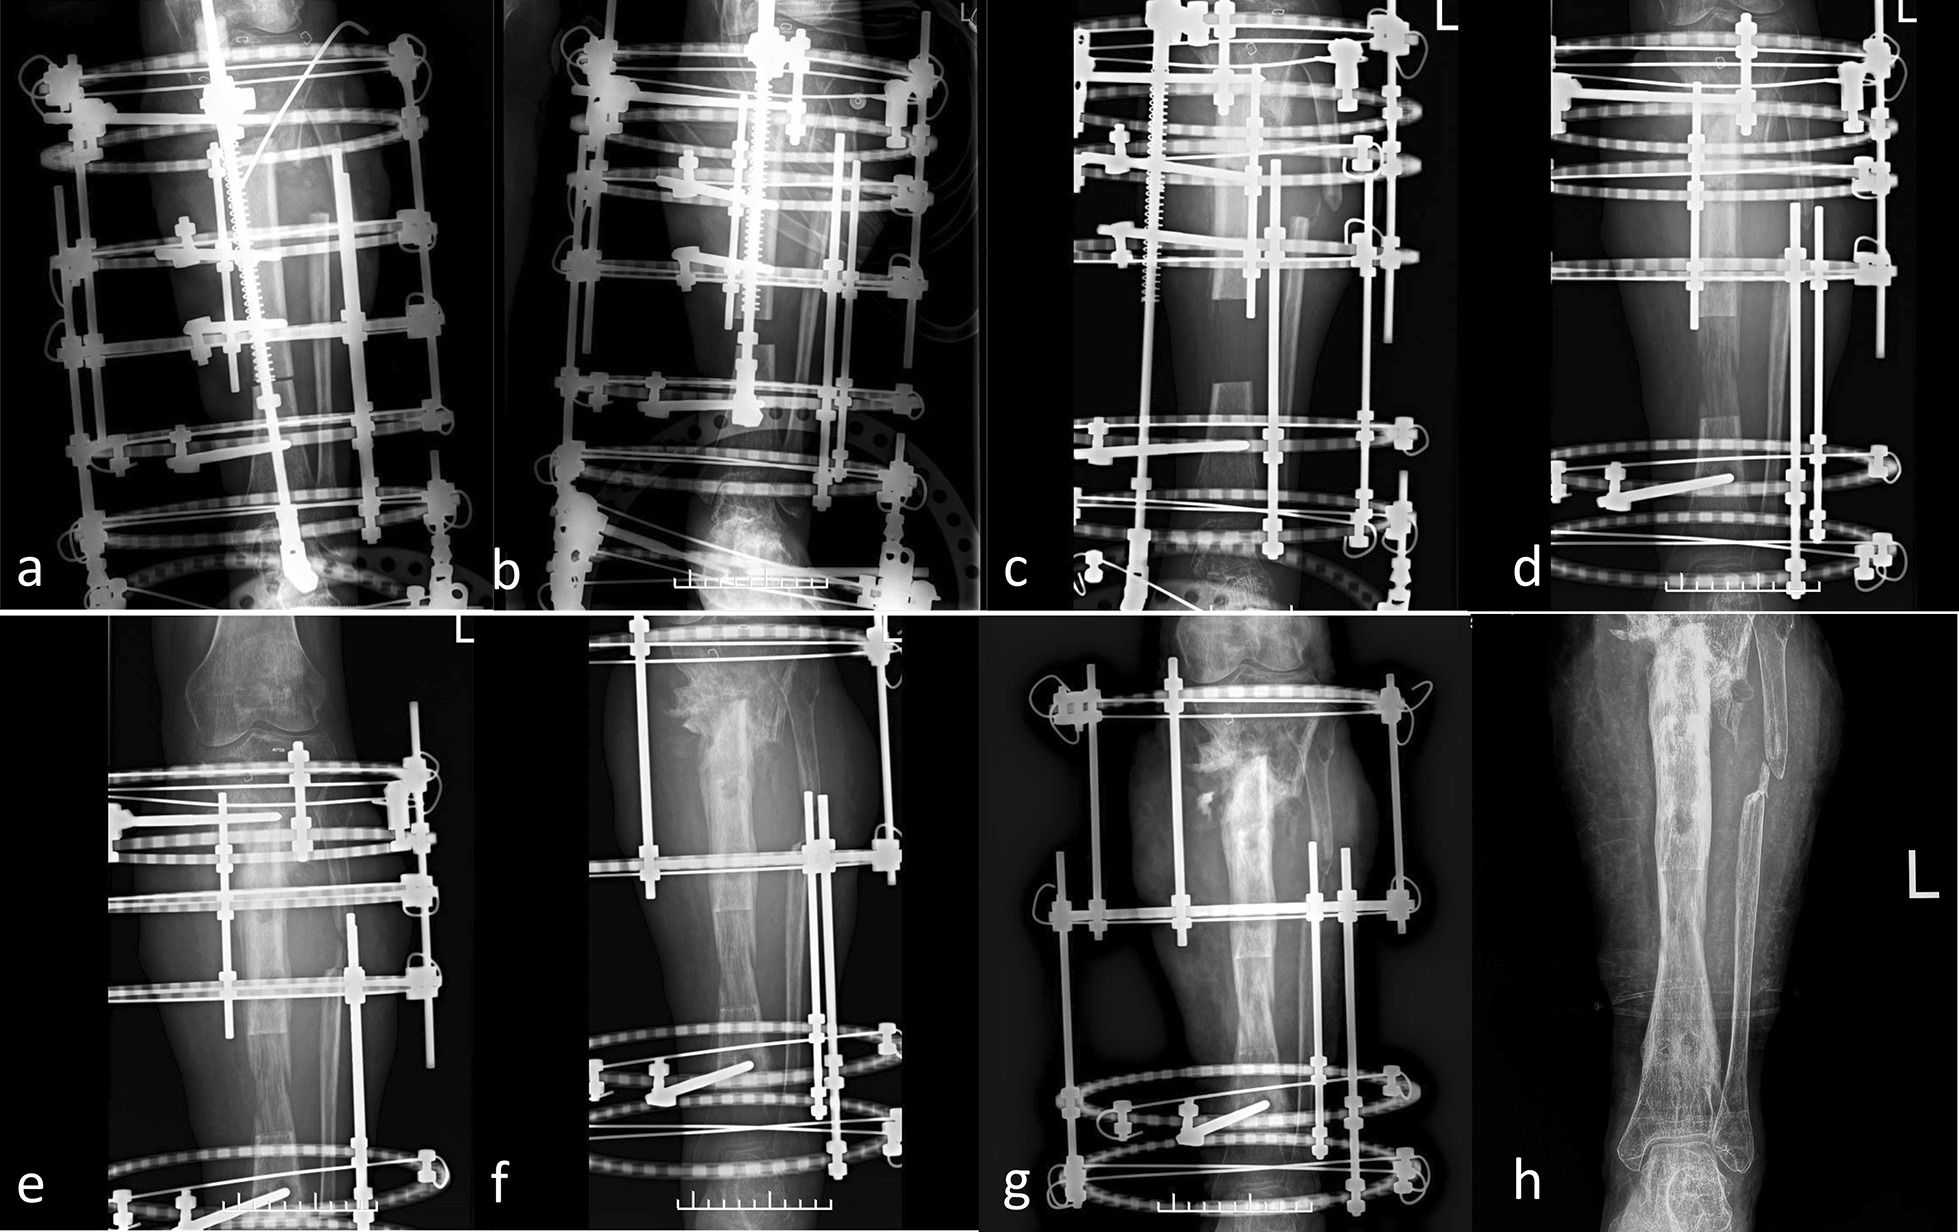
The bone transport process of a 50-year-old woman who had an infected left tibia bone defect with traditional Ilizarov technique. a Radiograph of a 50-year-old woman who had an infected left tibia bone defect with the start of transverse osteotomy. b Three weeks after operation. c Two months after operation. d–f Having the regenerated bone begun to be mineralized at the docking site at 7, 9 months and 1 year after operation. g Good consolidation and mineralization of the regenerated bone at 17 months after operation. h Completing bone union and removing the external fixation at 24 months after operation
